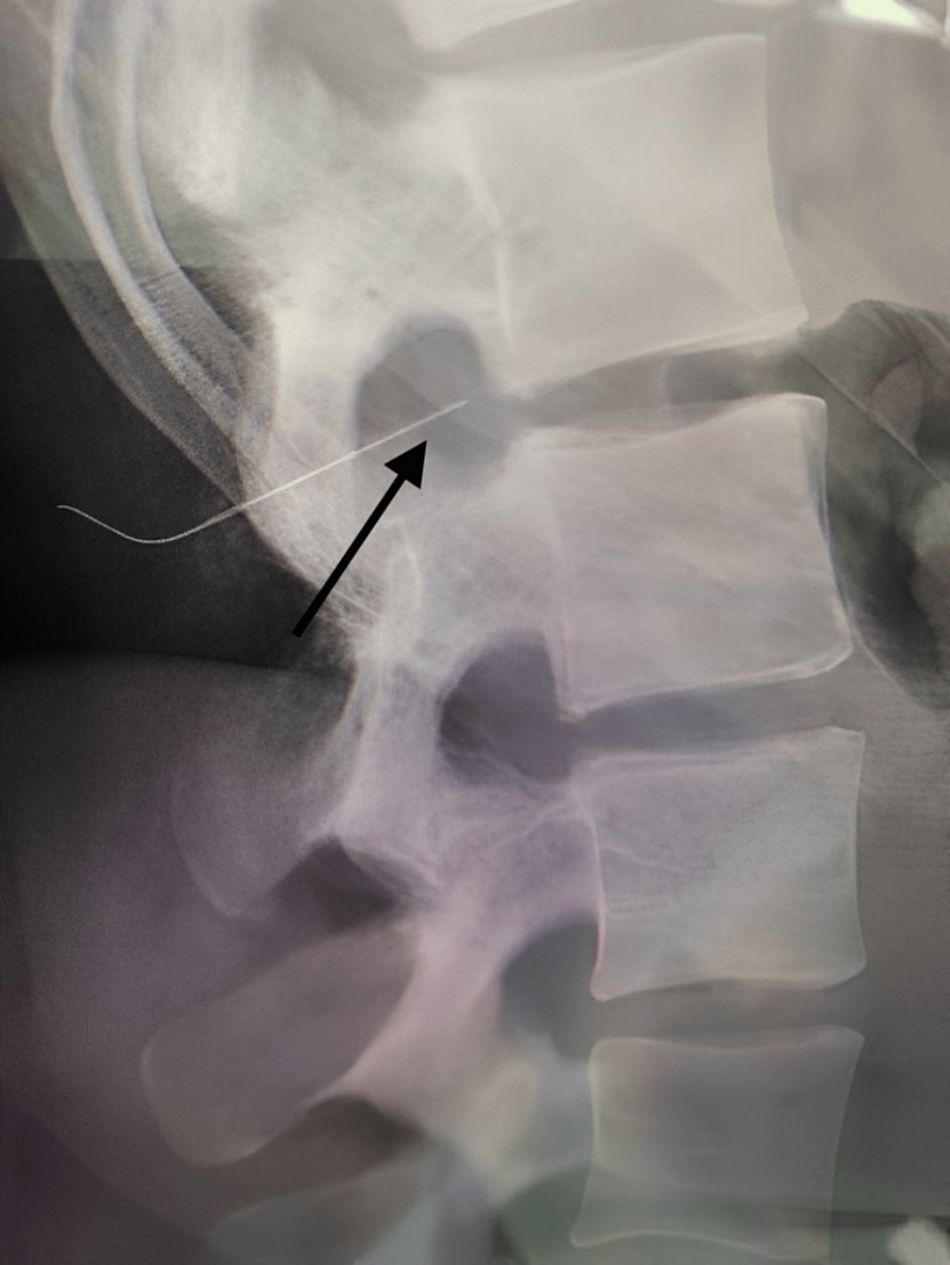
Lumbar spine X-ray lateral view showing needle tip opposite T12-L1
